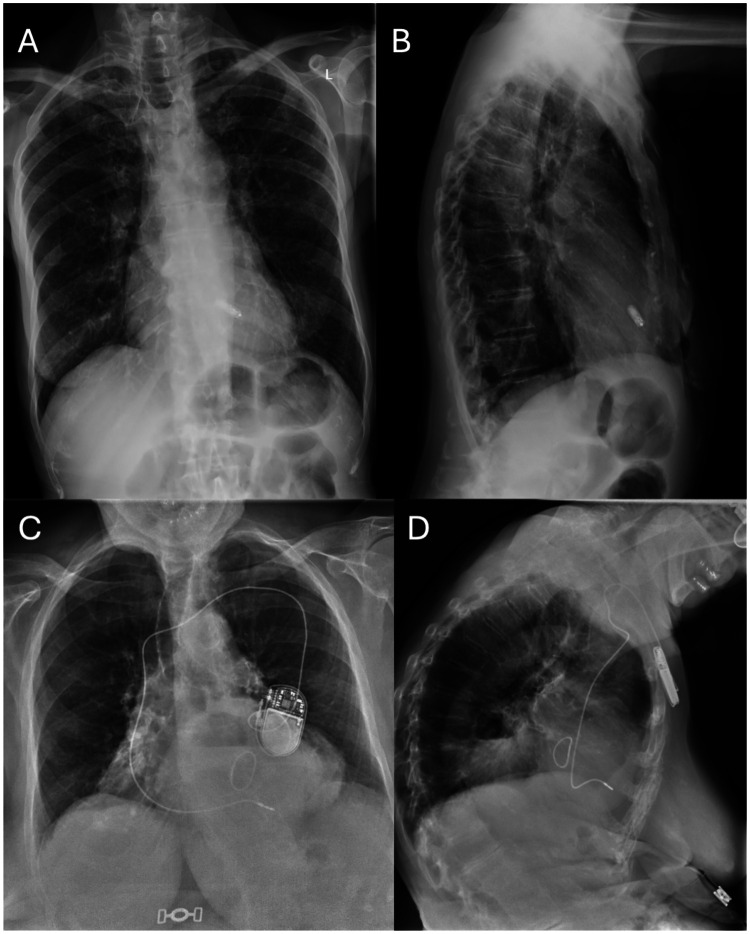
Chest x-Ray of patients with leadless pacemaker vs conventional single-chamber transvenous pacemaker. Chest x-Ray of patients with leadless pacemaker vs conventional single-chamber transvenous pacemaker. (A) Chest x-Ray of a patient with leadless pacemaker (postero-anterior view); (B) Chest x-Ray of a patient with leadless pacemaker (latero-lateral view); (C) Chest x-Ray of a patient with a conventional single-chamber transvenous pacemaker (postero-anterior view); (D) Chest x-Ray of a patient with a conventional single-chamber transvenous pacemaker (latero-lateral view).
